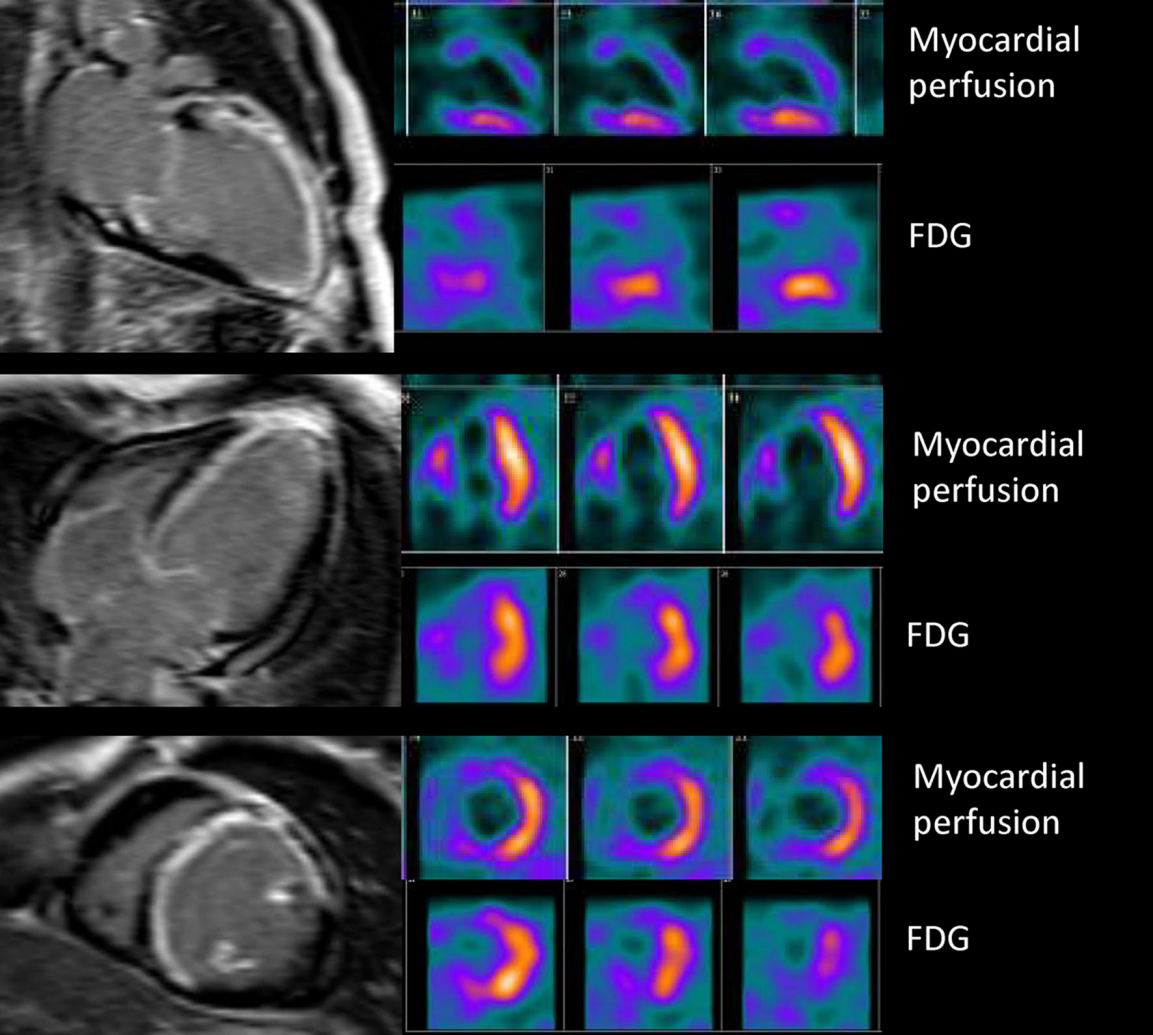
Myocardial scar and viability for assessment of risk of arrhythmic death. Example of a 57-year-old patient with anterior myocardial infarction and a left ventricular ejection fraction of 17%. Contrast-enhanced magnetic resonance images show large transmural scar in the anterior and septal walls and subendocardial (50% of the wall) in the inferoseptal wall. Selected vertical and horizontal long-axis and short-axis views of the left ventricle on ^99m^Technetium tetrofosmin SPECT show a perfusion defect of the septal, anterior and apical segments that match the defects on fluorine^18^-deoxyglucose SPECT. The patient received an implantable cardiac defibrillator (ICD) for primary prevention of sudden cardiac death. Two months later, the patient was admitted with an appropriate ICD shock
